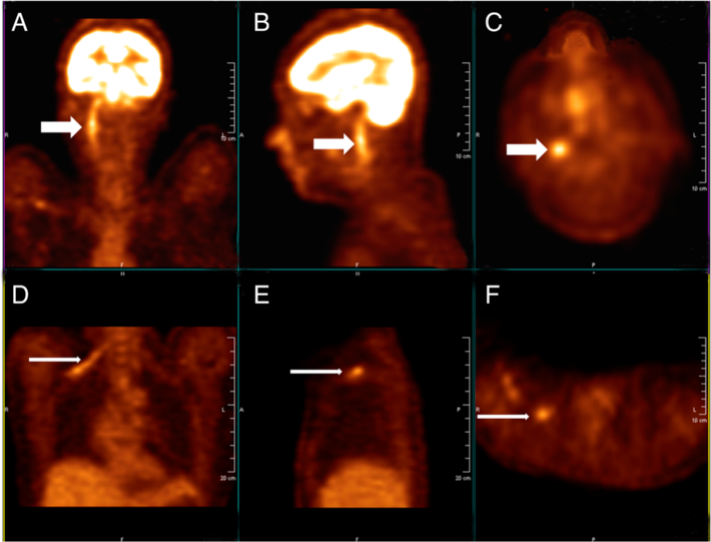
PET/CT images before treatment showing involvement of right vagus nerve and brachial plexus (detailed images). a, b, c show abnormally high glucose metabolism area of the right vagus nerve running zone; d, e, f show abnormally high glucose metabolism area of right brachial plexus running zone; a, d are coronal sections; b, e are sagittal sections; c,f are cross sections; white arrows direct at lesions in nerves
